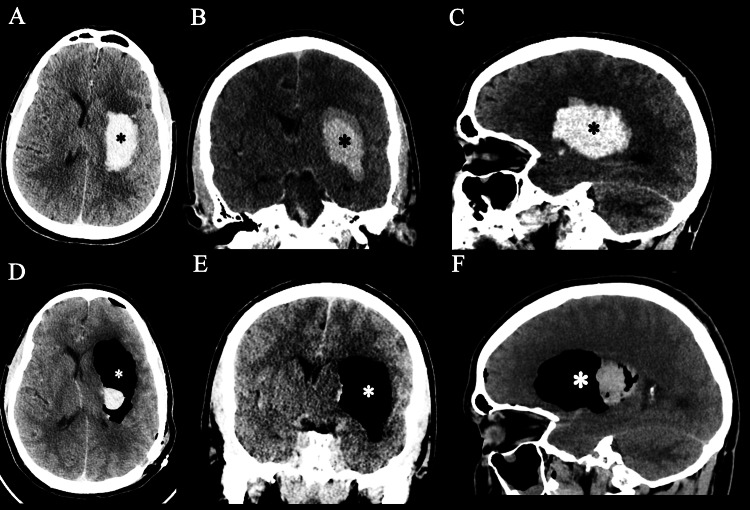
Preoperative CT brain images (A, B, C) showing a large basal ganglia hemorrhage (*) involving the globus pallidus and the internal capsule, and postoperative CT brain images (D, E, F) showing the nearly complete evacuation of the hematoma with the space being replaced with pneumocephalus (*) and a small residual hematoma
